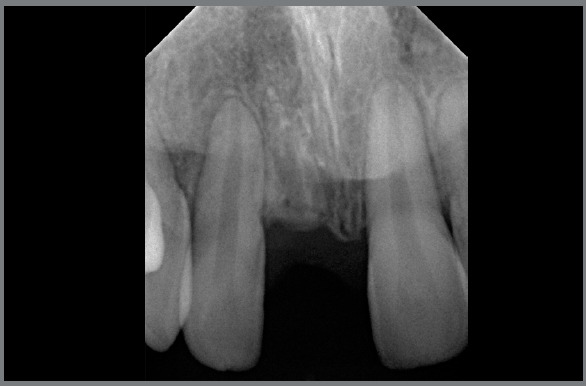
Radiographic aspects after eight months of decoronation.
